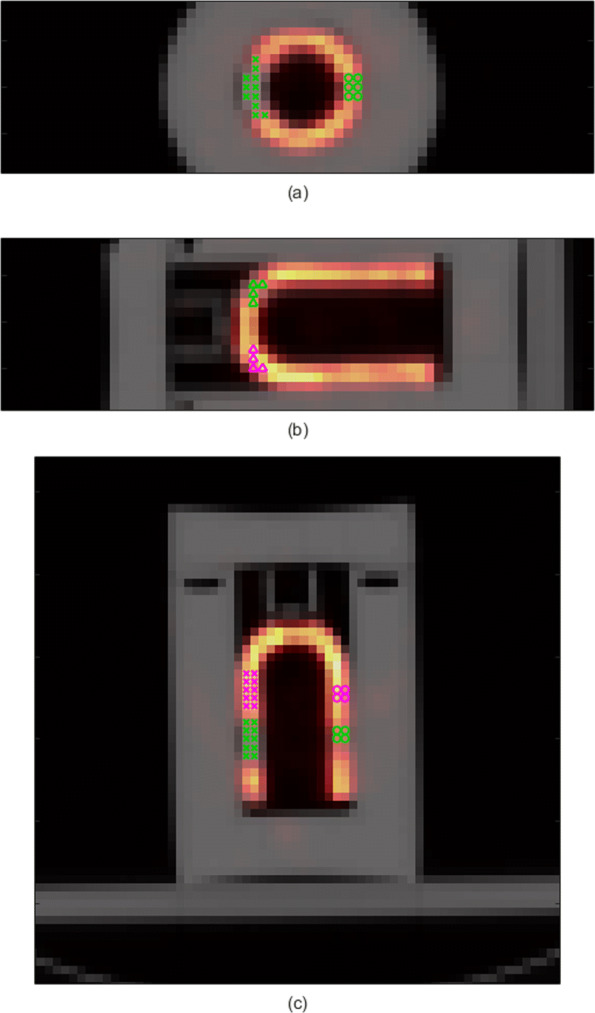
Defect and normal myocardial regions. Stationary SPECT image fused with CT image is displayed. Coronal (a), sagittal (b), and transaxial (c) views are displayed. The defect regions are displayed with green markers and the normal myocardial regions (references) with purple markers. The large segmental defect is displayed with crosses (×; in a and c) and the two smaller, cubical defects are displayed with circles (*o*; in a and c) and triangles (*Δ*; in b)
